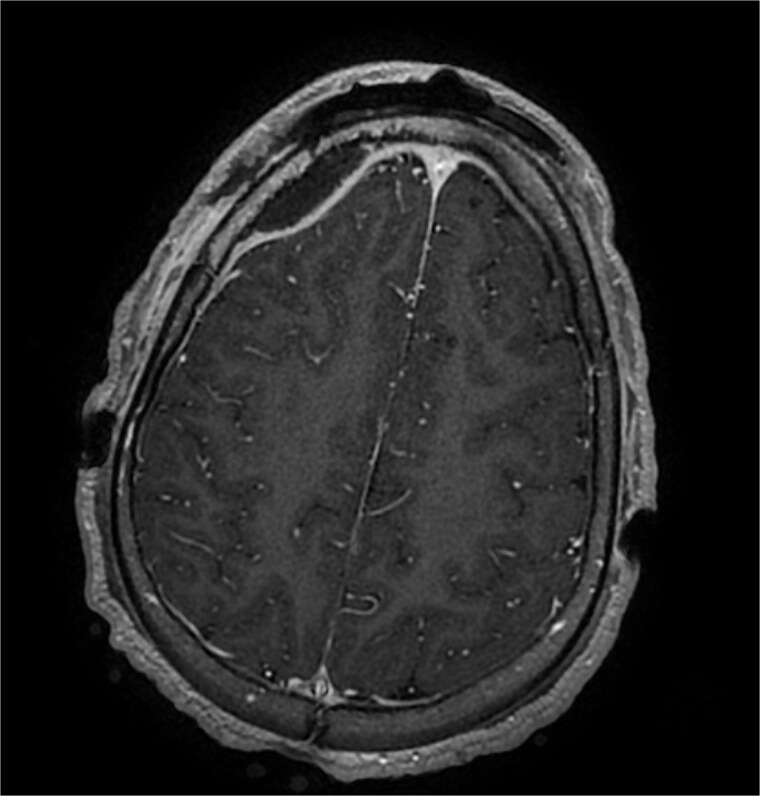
Axial contrast-enhanced T1-weighted MRI at postoperative day 10 showing persistent cerebral empyema.
